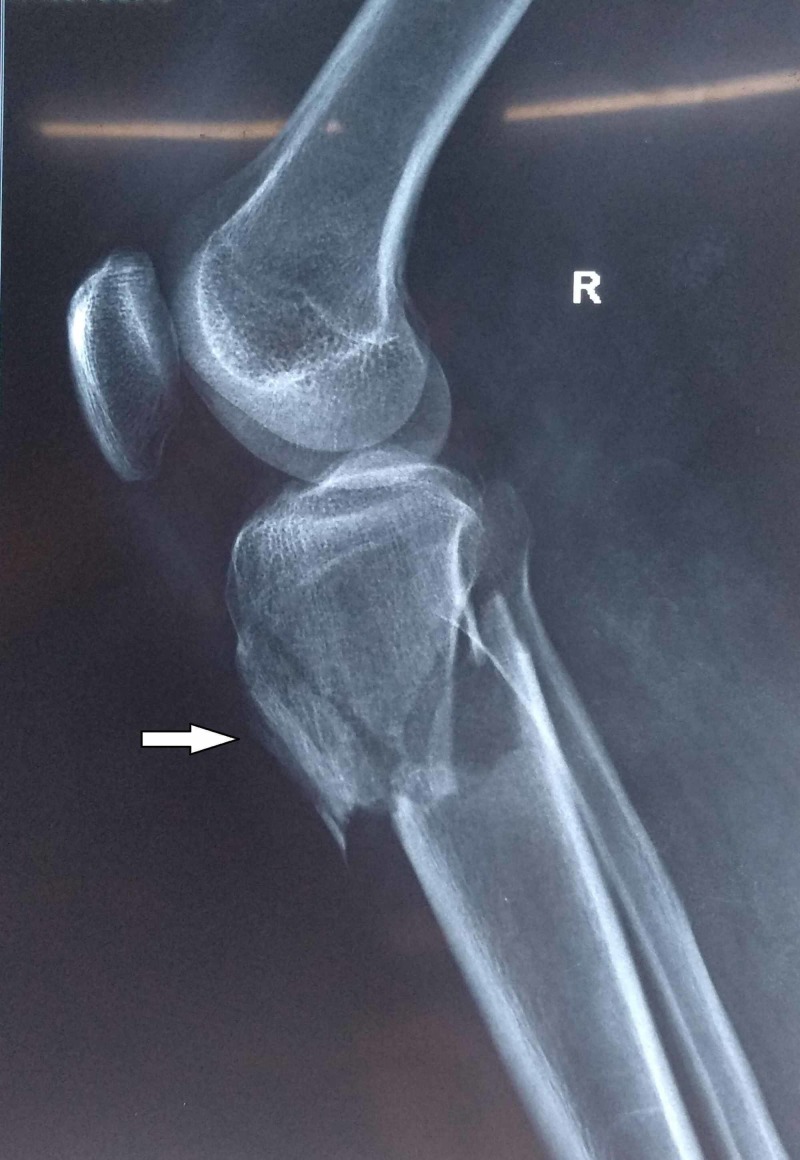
Tibial plateau fracture with tibial tuberosity avulsion fracture radiograph (white arrow)
